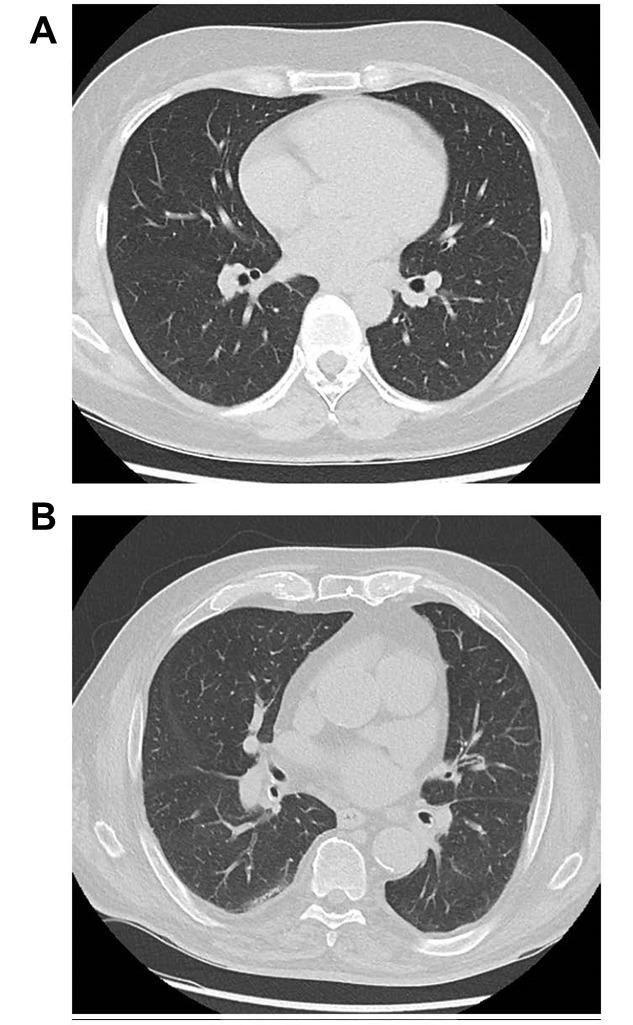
Computed tomography scan (CT) of the lungs. (A) healthy young individual; (B) discrete subpleural reticulations in the dorsal lung segments of the right lower lobe in an asymptomatic 85-year-old patient.
